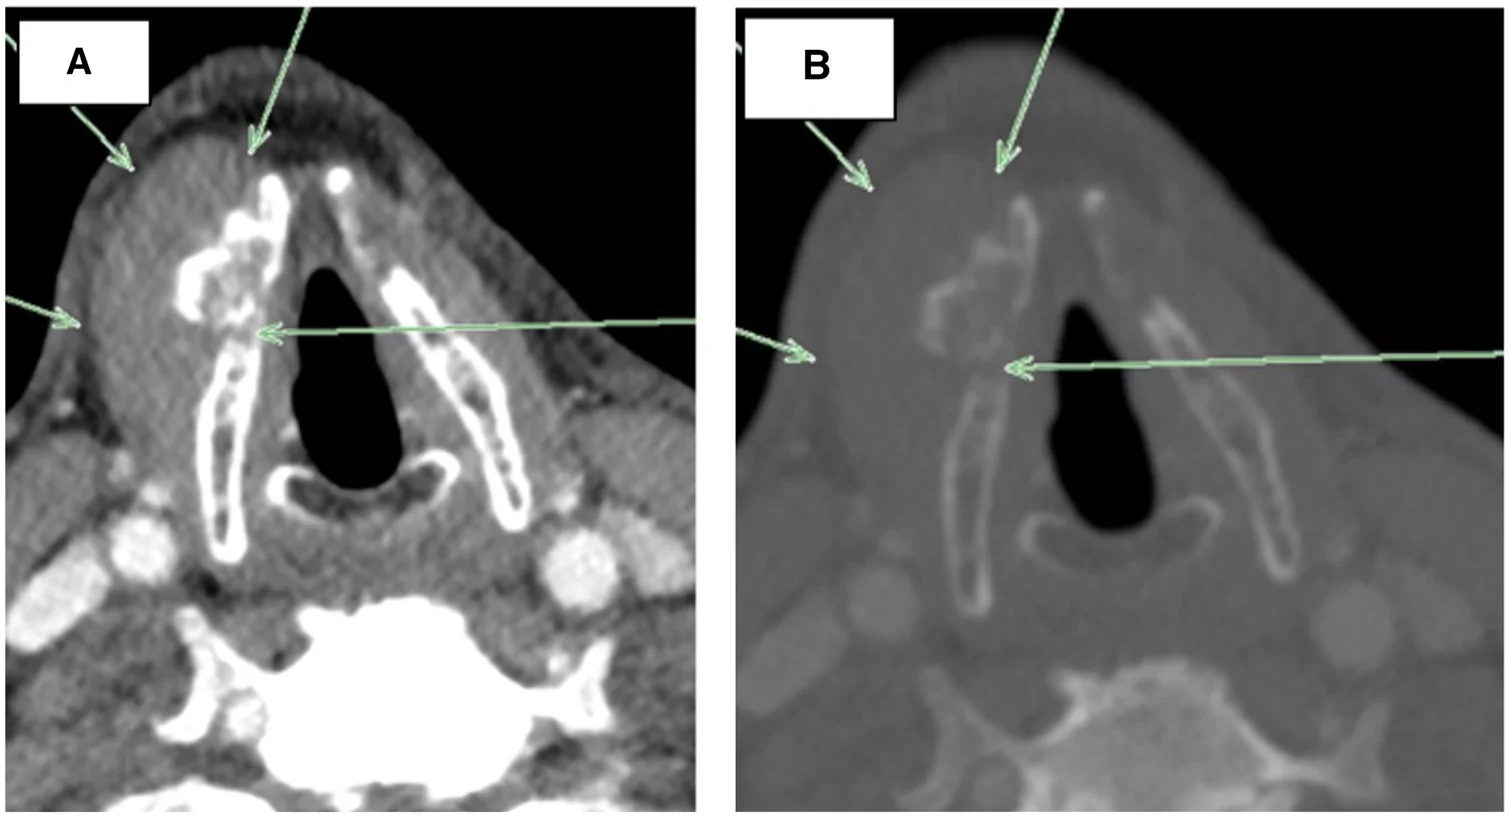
(A) Contrast-enhanced CT (soft tissue window) of the neck. Axial CT demonstrates an exophytic, well-defined, encapsulated soft tissue density mass arising from the anterosuperior aspect of the right thyroid cartilage lamina, containing foci of chondroid matrix mineralisation. There is no extension into the paraglottic space or laryngeal vestibule, and no evidence of aggressive soft tissue infiltration. (B) Contrast-enhanced CT (bone window) of the neck. Bone window CT further demonstrates the well-circumscribed exophytic lesion arising from the right thyroid cartilage lamina, with internal matrix calcification and no features of cortical destruction or aggressive bony erosion.
